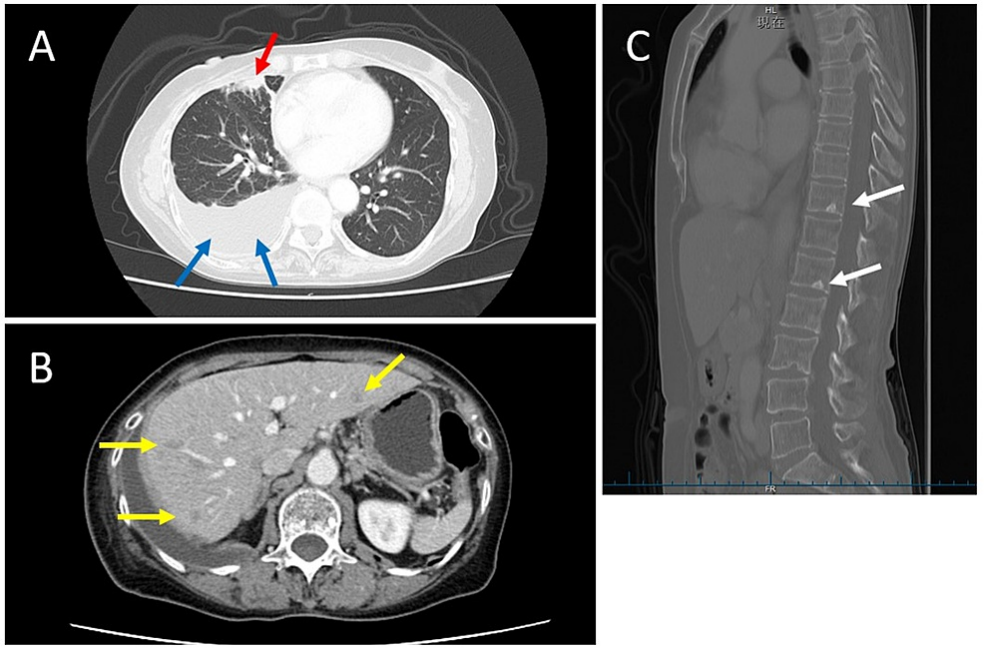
CT scan images. (A) The red arrow indicates lung metastasis, and the blue arrows indicate pleural effusion. (B) Yellow arrows indicate multiple liver metastases. (C) The white arrows indicate multiple bone metastases. CT: computed tomography
